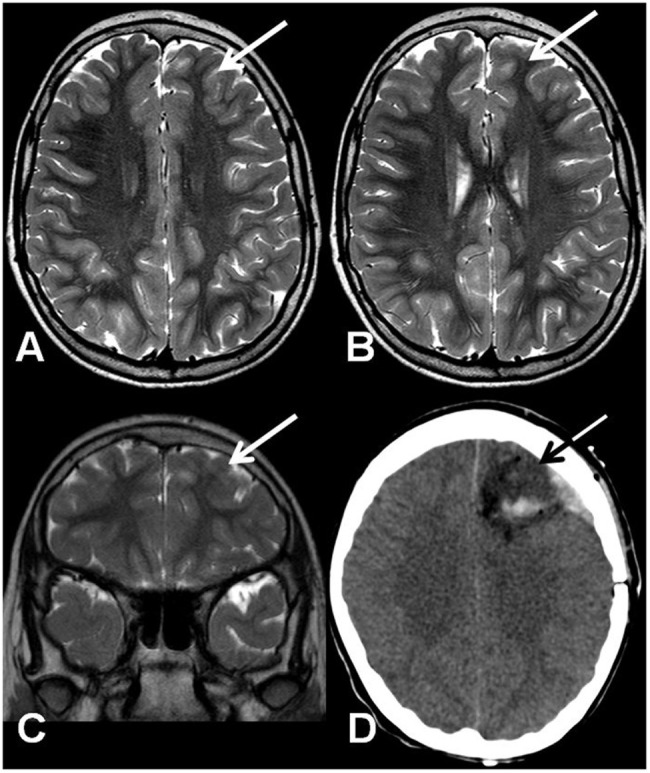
(A,B) Axial T2-weighted TSE MRI. Coronal T2-weighted TSE MRI (C) and axial computed tomography after surgery (D). The arrows show a mildly hypoplastic frontal lobe with focal T2 signal hyperintensity on the cortico-subcortical transition (A–C) and the resected region (D).
